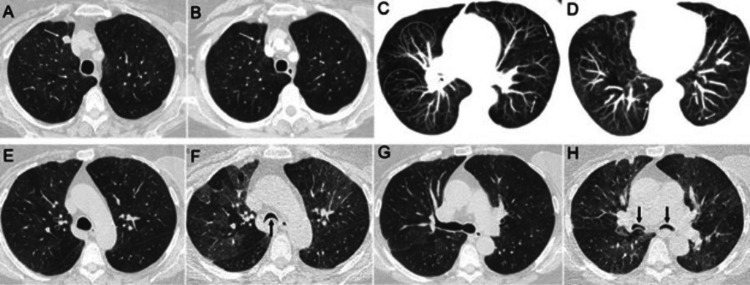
Diffuse idiopathic pulmonary neuroendocrine cell hyperplasia with a carcinoid tumor. Axial CT image (A) and a corresponding axial image from a CT performed nine years earlier (B) demonstrate an indolent interval enlargement of a right upper lobe nodule (arrows). Axial maximum intensity projection images (C and D) reveal numerous small lung nodules (<6 mm), some marked by arrows or dashed circles; most of these nodules remained stable over several years. During inspiration scans (E and G), mosaic attenuation is evident, while expiration scans (F and H) show patchy expiratory air trapping along with excessive dynamic airway collapse of the trachea and main bronchi (arrows). Surgical resection confirmed the presence of a carcinoid tumor in the right upper lobe nodule, along with adjacent carcinoid tumorlets.
